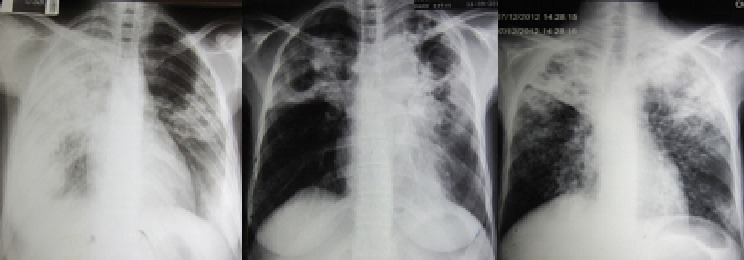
Radiographies thoraciques montrant des PT bilatérales
